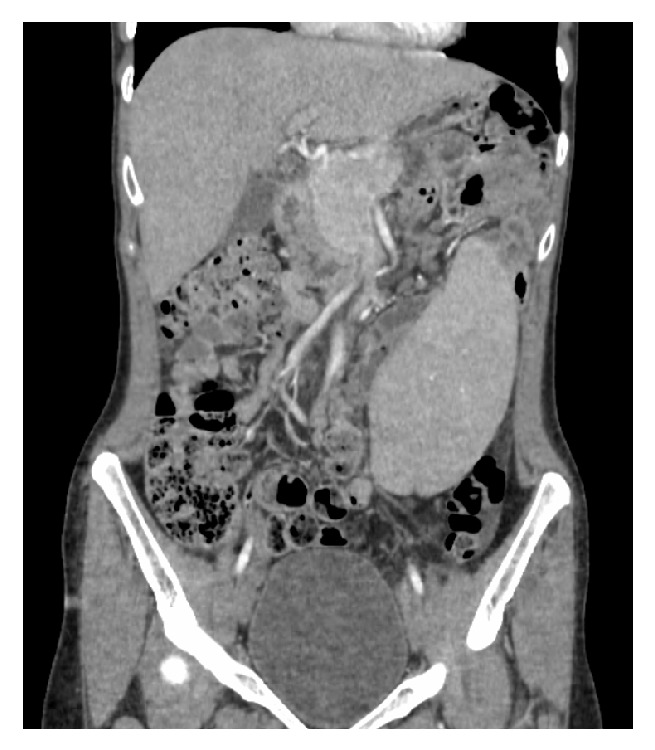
CT of the abdomen demonstrating spleen in the inferior quadrant of the abdomen.
